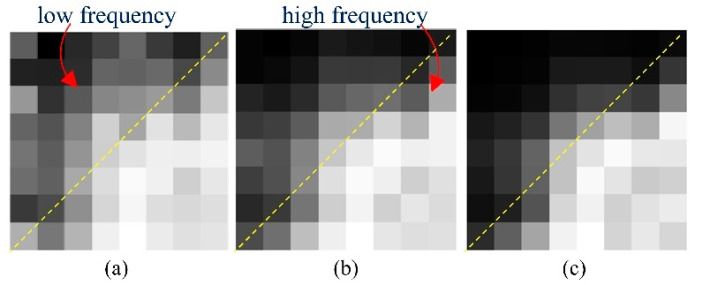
Average reconstruction error of 64 DCT frequencies with different degradation levels. Each pixel represents the MSE between FBP reconstruction and the ideal CT image at the corresponding frequency. The 300 evaluated images were selected from The Cancer Imaging Archive [29,30] for different body parts: (a) reconstruction error of 60 views; (b) reconstruction error of 120 views; and (c) reconstruction error of 240 views.
